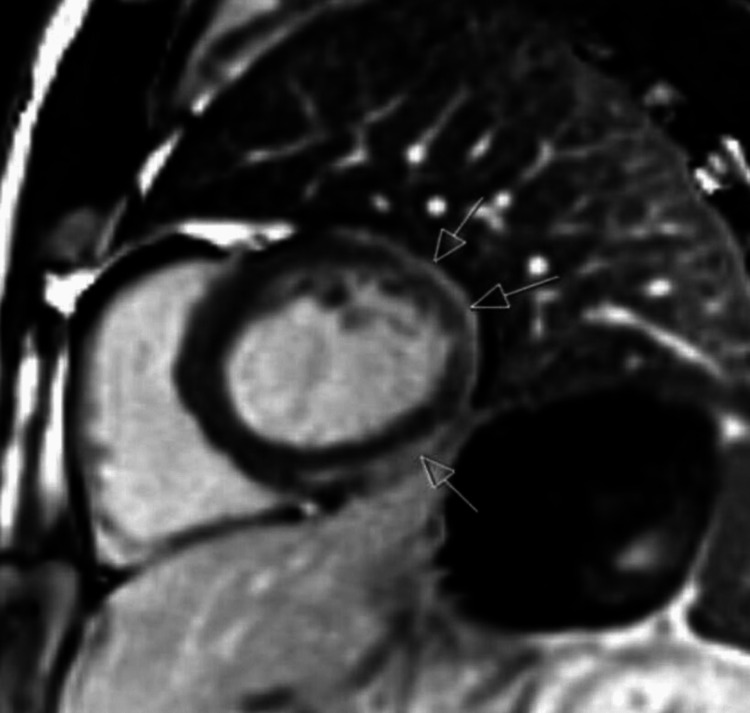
Cardiovascular magnetic resonance imaging (CMR) short axis view showing extensive, crescentic-shaped, subepicardial late gadolinium enhancement from basal to apical inferior, basal to apical lateral, and mid to apical anterior segments as shown by arrows.
